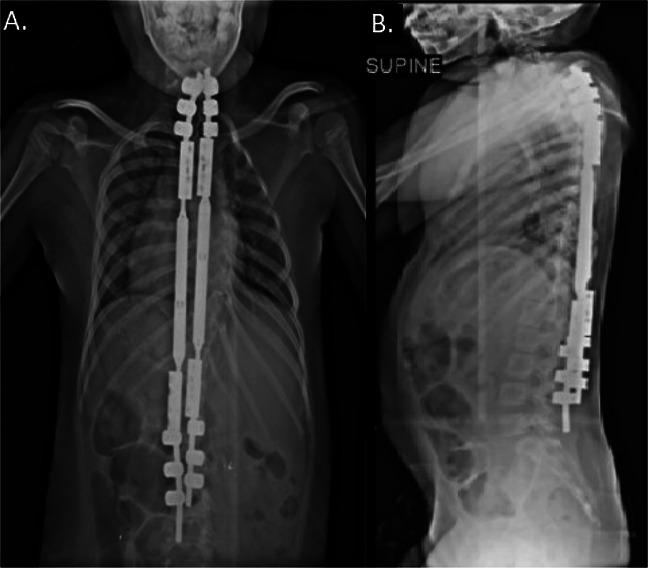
Radiographic images of the patient after MAGEC rod exchange. A, PA view. B, Lateral view.
